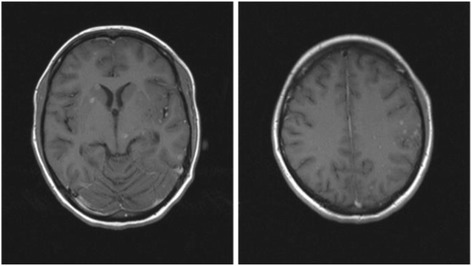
Second magnetic resonance imaging brain images showing regression of the size and number of intracerebral tuberculomas
